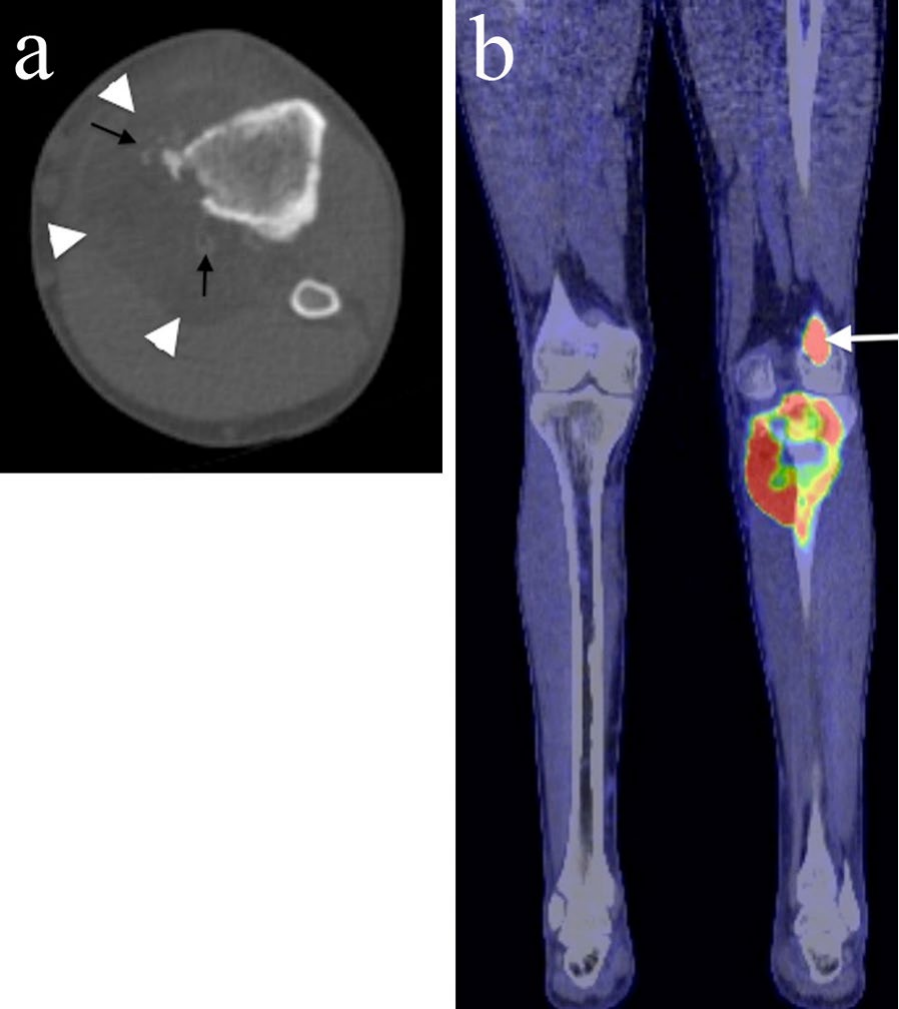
Axial CT and PET/CT imaging of the proximal tibial lesion. a Axial CT imaging shows medial cortical destruction and a large soft tissue mass containing areas of fat attenuation (white arrowheads) and ossification (black arrows). b A coronal fused PET/CT image shows there is marked FDG uptake in the proximal tibial tumour as well as in a lateral distal femoral metastasis (white arrow). No other lipomatous lesion or tumour is present
